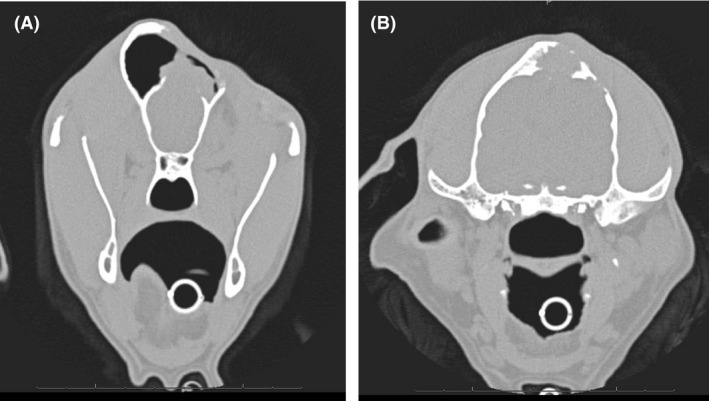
Computed tomography. Transversal plane. 12 month after surgery. (A) Soft tissue mass in the frontal sinus. (B) Destruction of the cranial bone.
